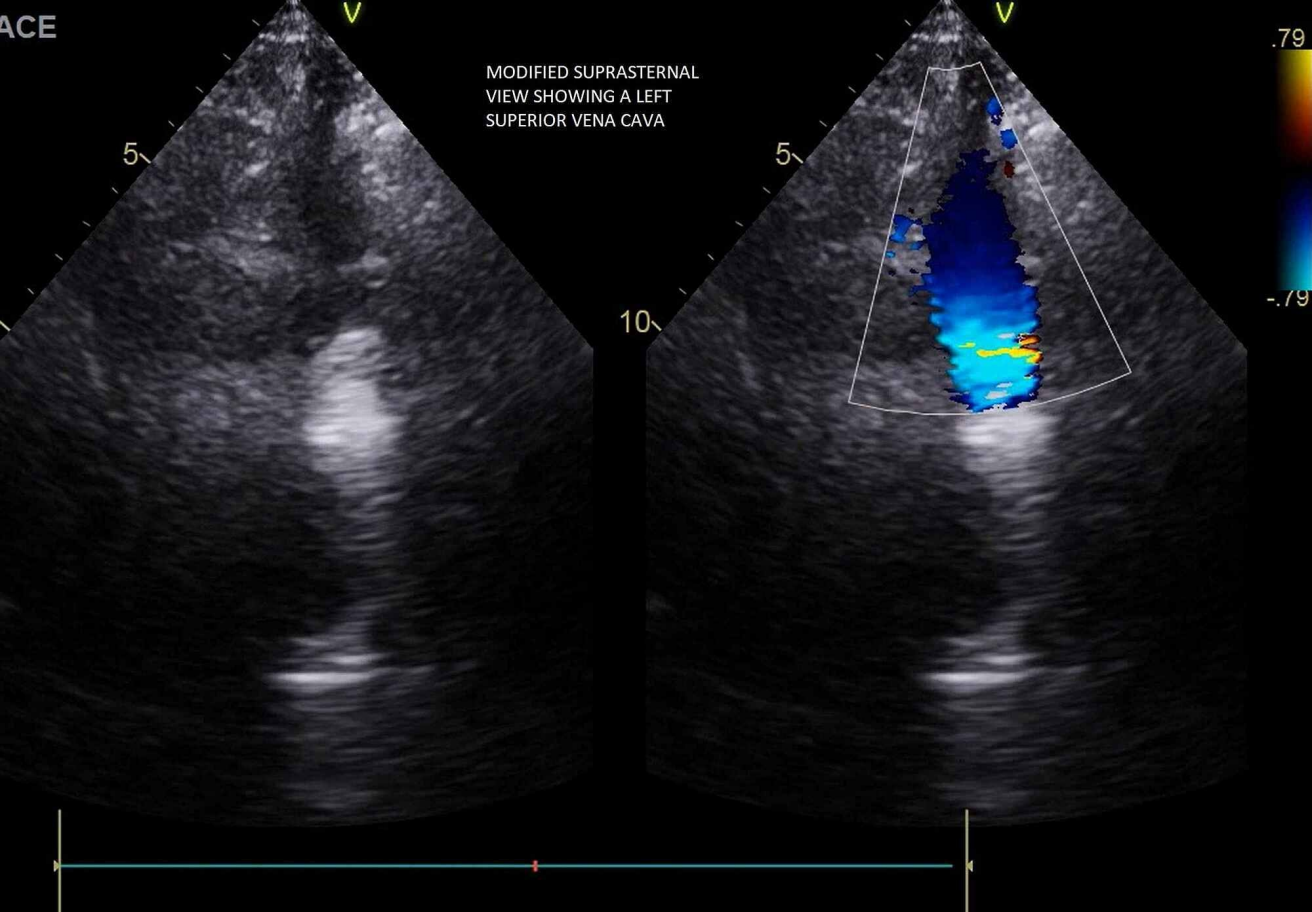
Modified suprasternal view with color Doppler showing a persistent left superior vena cava
